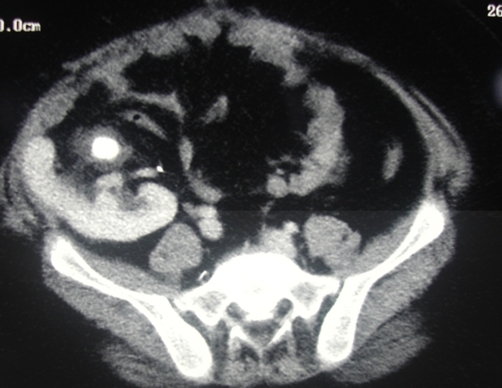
CT showing an inversely placed kidney with stone in the renal pelvis.
